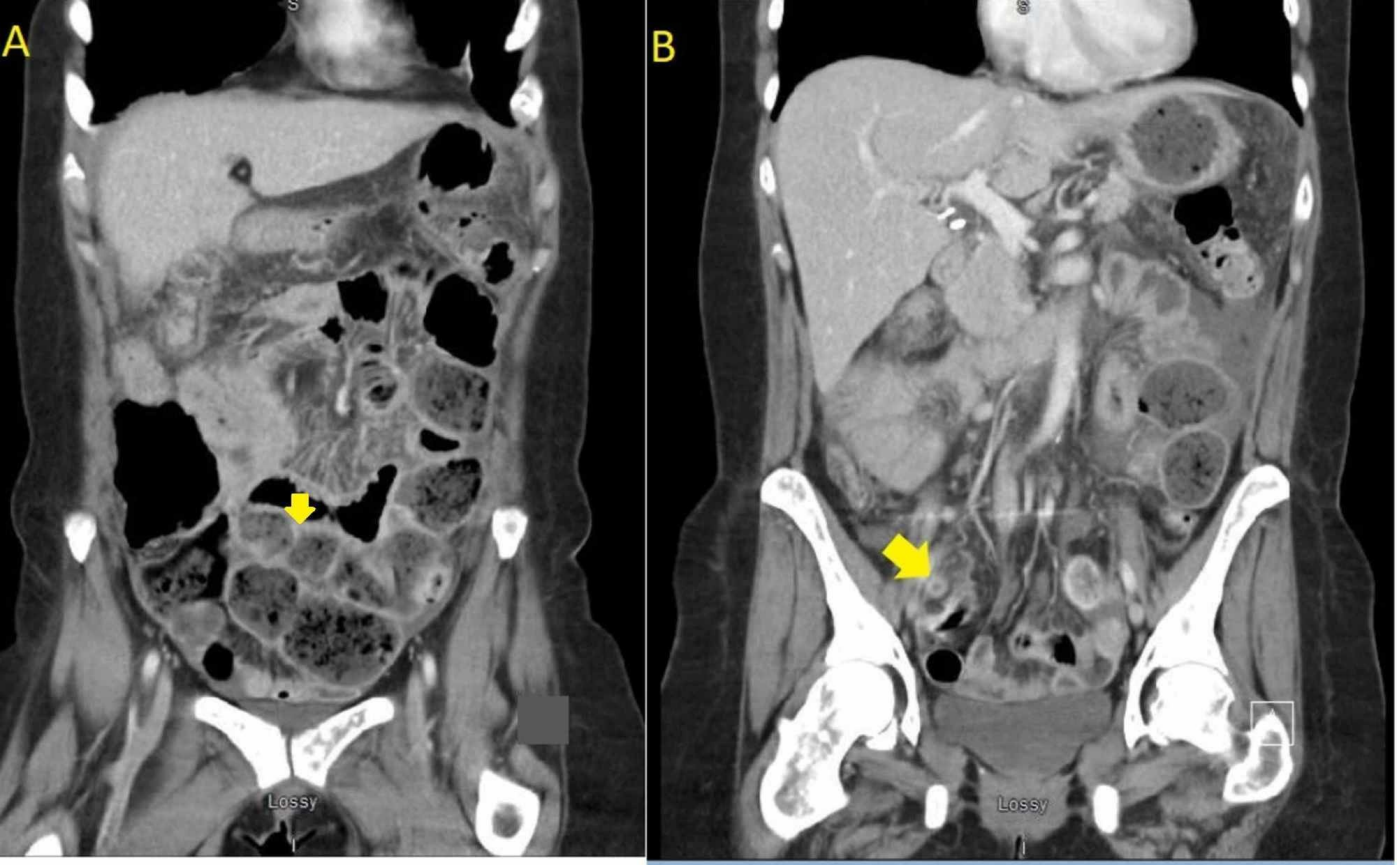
Coronal sections of the CT scan of the abdomen and pelvis. A: Showing dilated bowel lobes suggesting intestinal obstruction; B: Arrow pointing at the swollen appendix. CT: Computed Tomography
